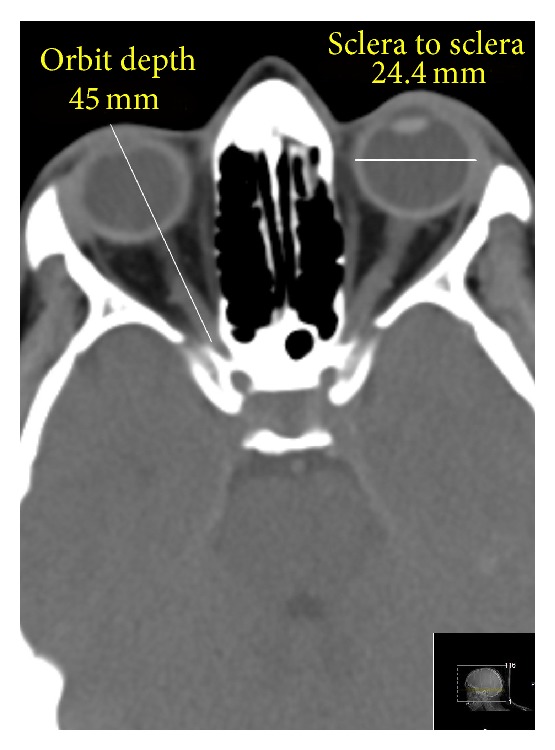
The retina to retina transverse diameter of the eye measured by computed tomography.
